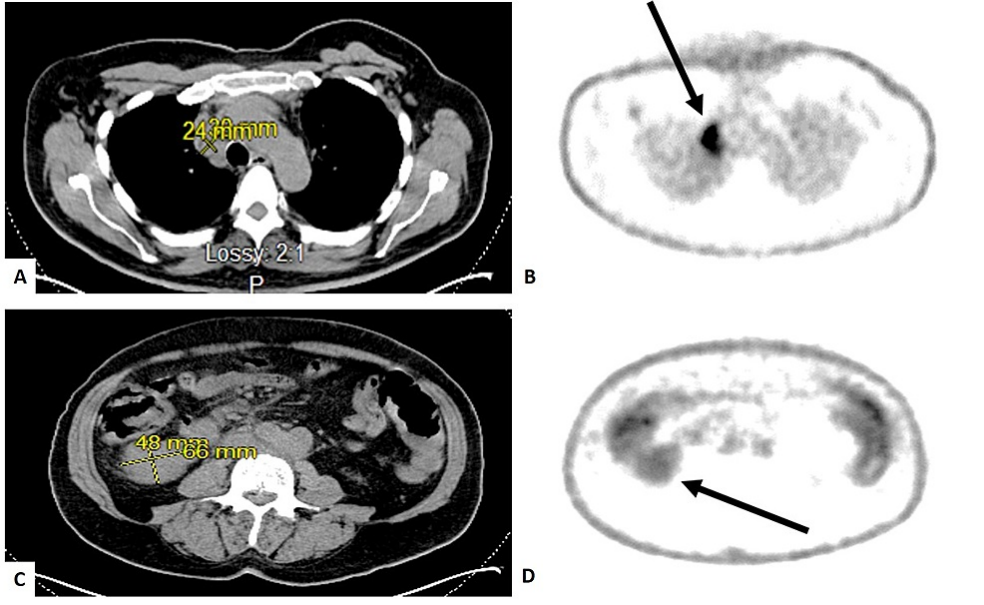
CT and corresponding PET scan demonstrating the PET avidity (arrows) in the enlarged lymph nodes. The transverse plane demonstrates PET avid enlarged mediastinal lymph node (A, B) and lymph node in the right lower quadrant (C, D). PET: positron emission tomography
